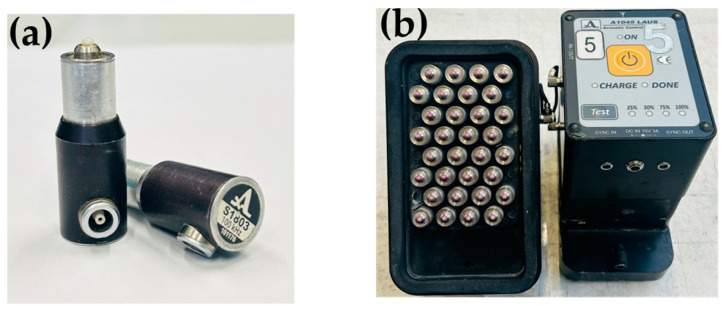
(a) Single DPC shear wave transducer 50 kHz center frequency [13], (b) single ultrasound unit consisting of 32 DPC transducers.
